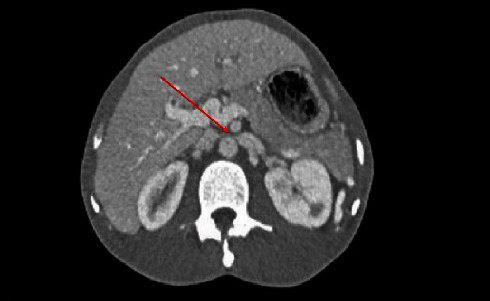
CT scan reveals a dilated left renal vein entrapped between the SMA and aorta with an arrow pointing to the compression area. CT, computed tomography. SMA, superior mesenteric artery.
